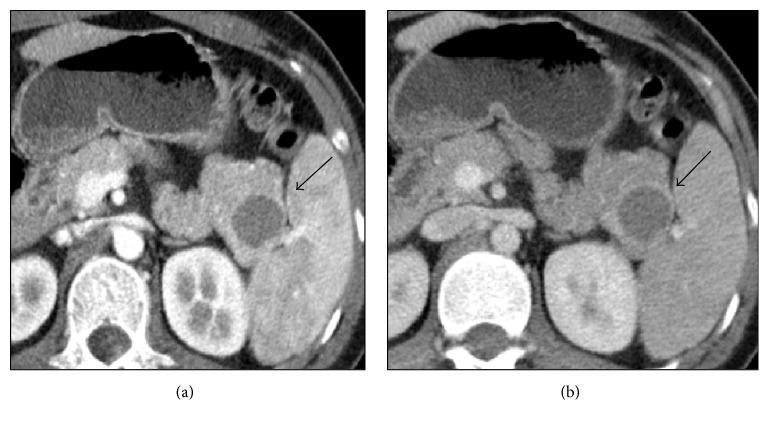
(a) Arterial and (b) portal-venous phase CT, confirming cystic lesion in pancreatic tail without soft-tissue component.
